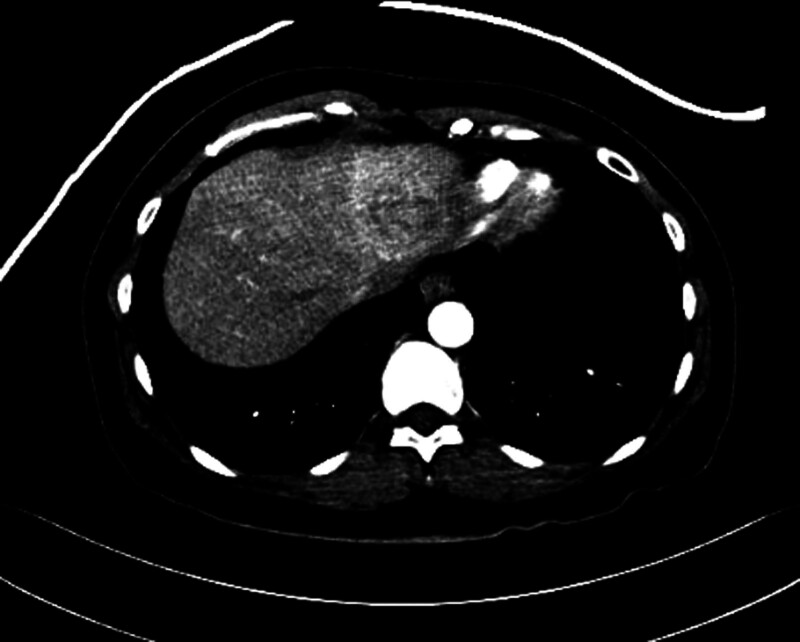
Abdominal and pelvic CT 1 mo after radiotherapy. Abdominal computed tomography revealed a 4 cm ill-defined, heterogeneously enhancing lesion in the superior portion of the left liver, suggestive of metastasis. CT = computed tomography.
